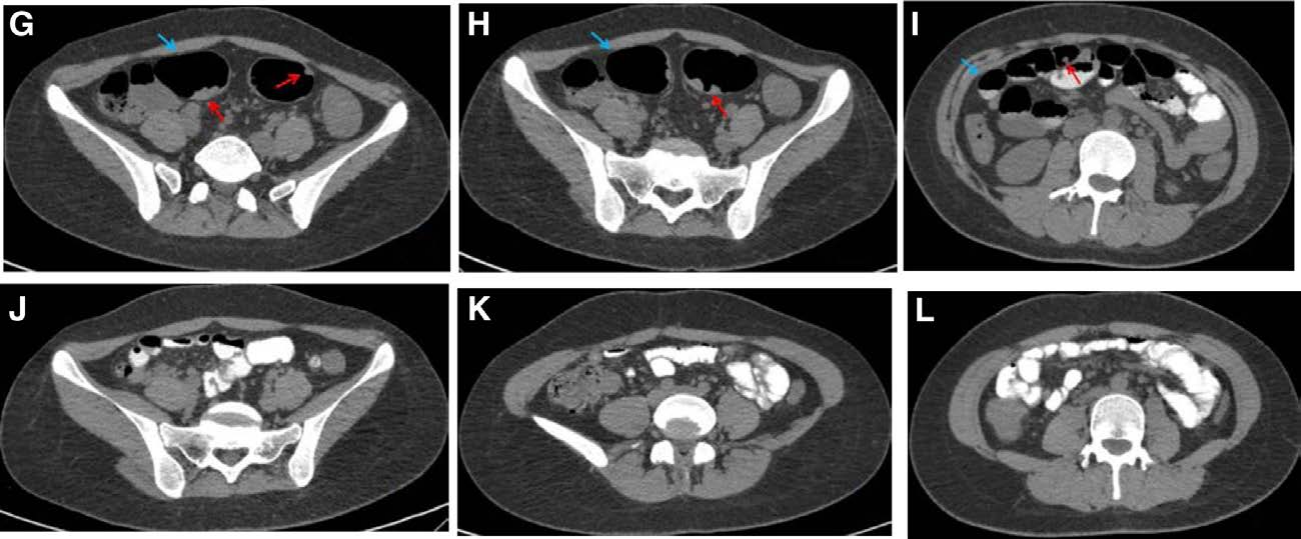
Image g, h and i indicates that abdominal CT before treatment showed significant intestinal dilatation (blue arrow), multiple polyps in colon (red arrow), and Image j, k and l indicates that no significant abnormalities were observed in CT after treatment.
